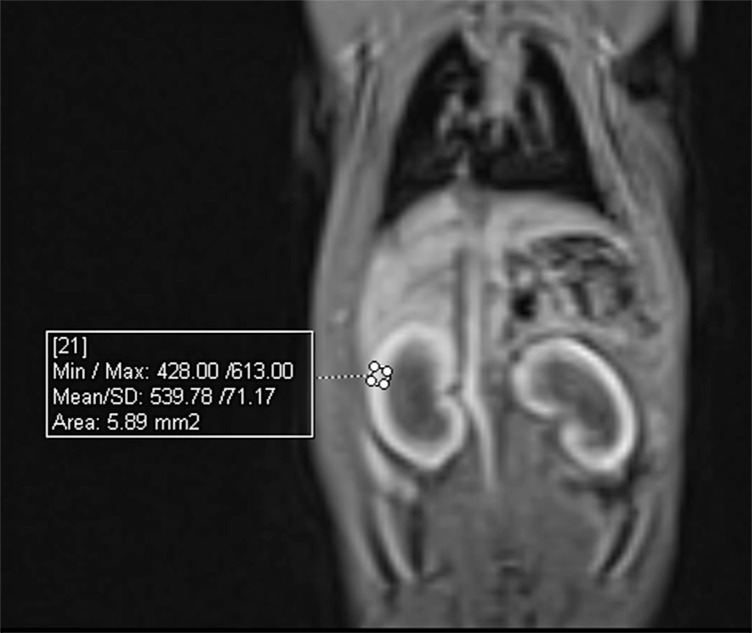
Coronal T1-weighted, contrast-enhanced image showing the regions of interest method for the measurement of renal parenchymal signal intensity.
